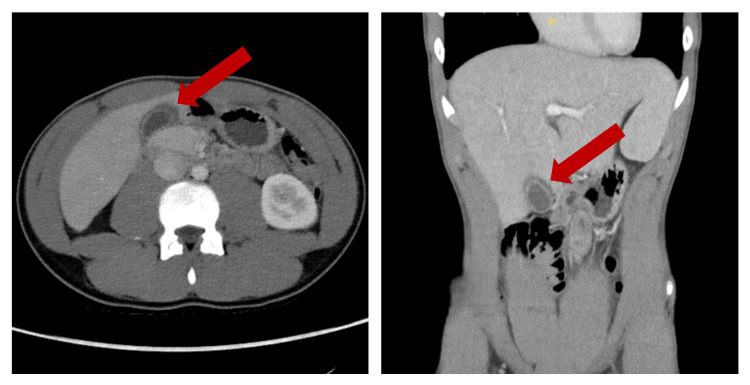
Initial CT abdomen and pelvis with nonspecific pericholecystic fluid
